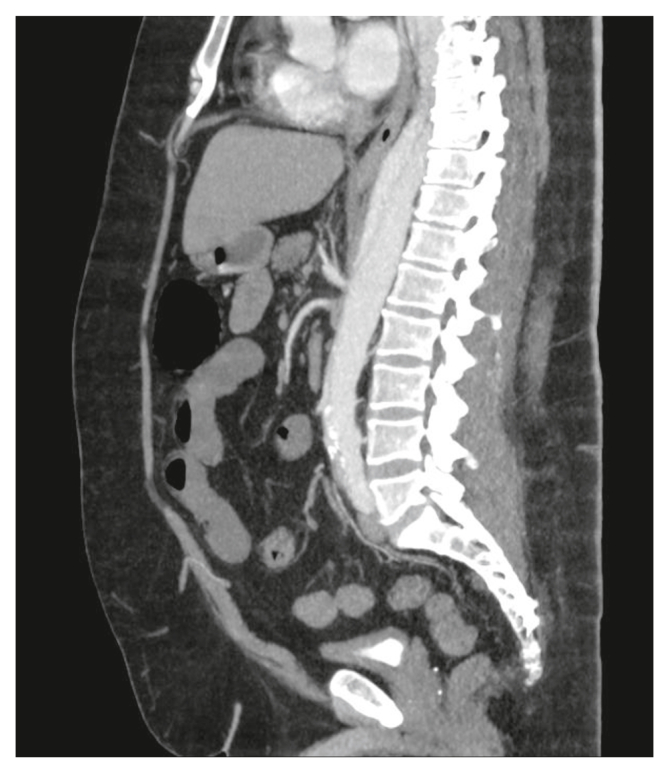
Abdominal computed tomography angiography showing a complicated type B aortic dissection of the thoracic and abdominal aorta and a dissection of the superior mesenteric artery with pre-existing atherosclerotic plaques.
